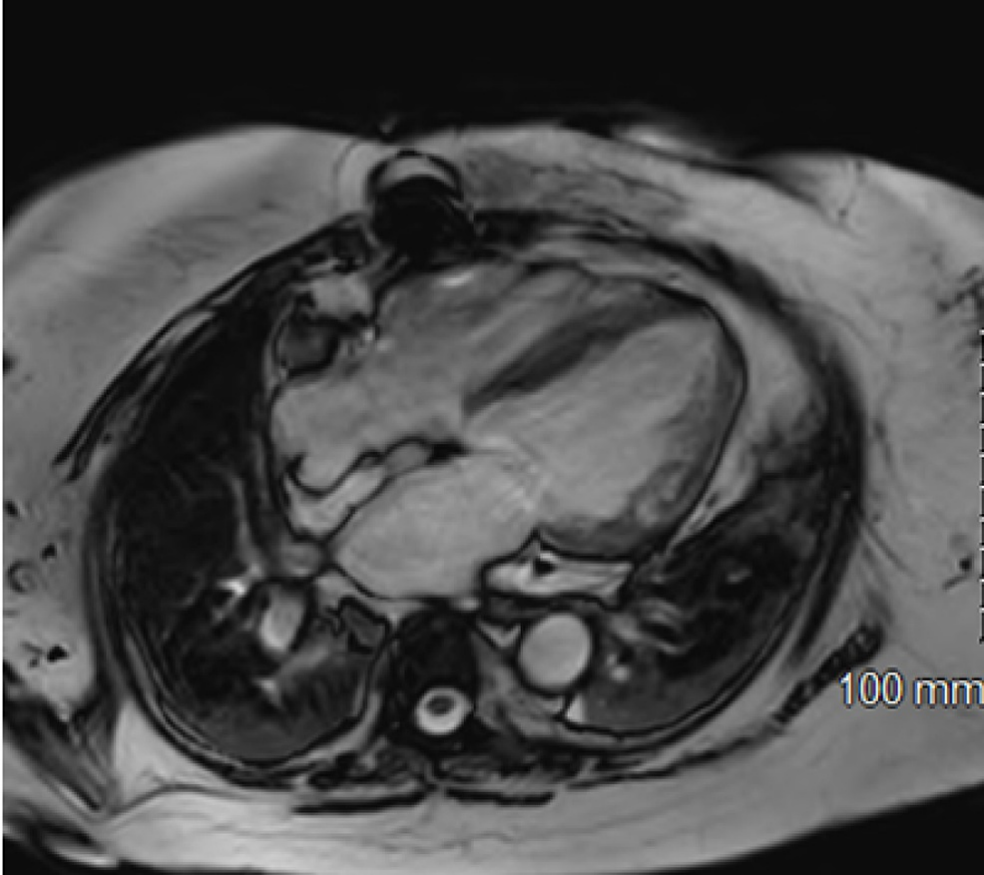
Cardiac MRI Combination of transmural and subendocardial enhancement involving the septal wall extending into the inferior septal region
